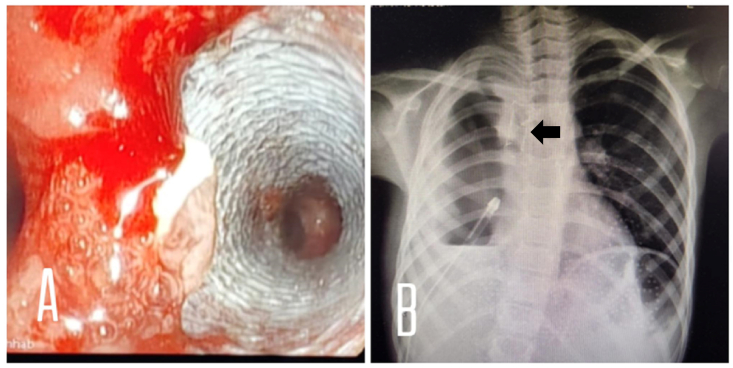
Bronchoscopic view (A) and chest X-ray (CXR) view (B) of a fully covered self-expandable metal stent (FC-SEMS) placed in right main bronchus (pointed by an arrow in CXR).
